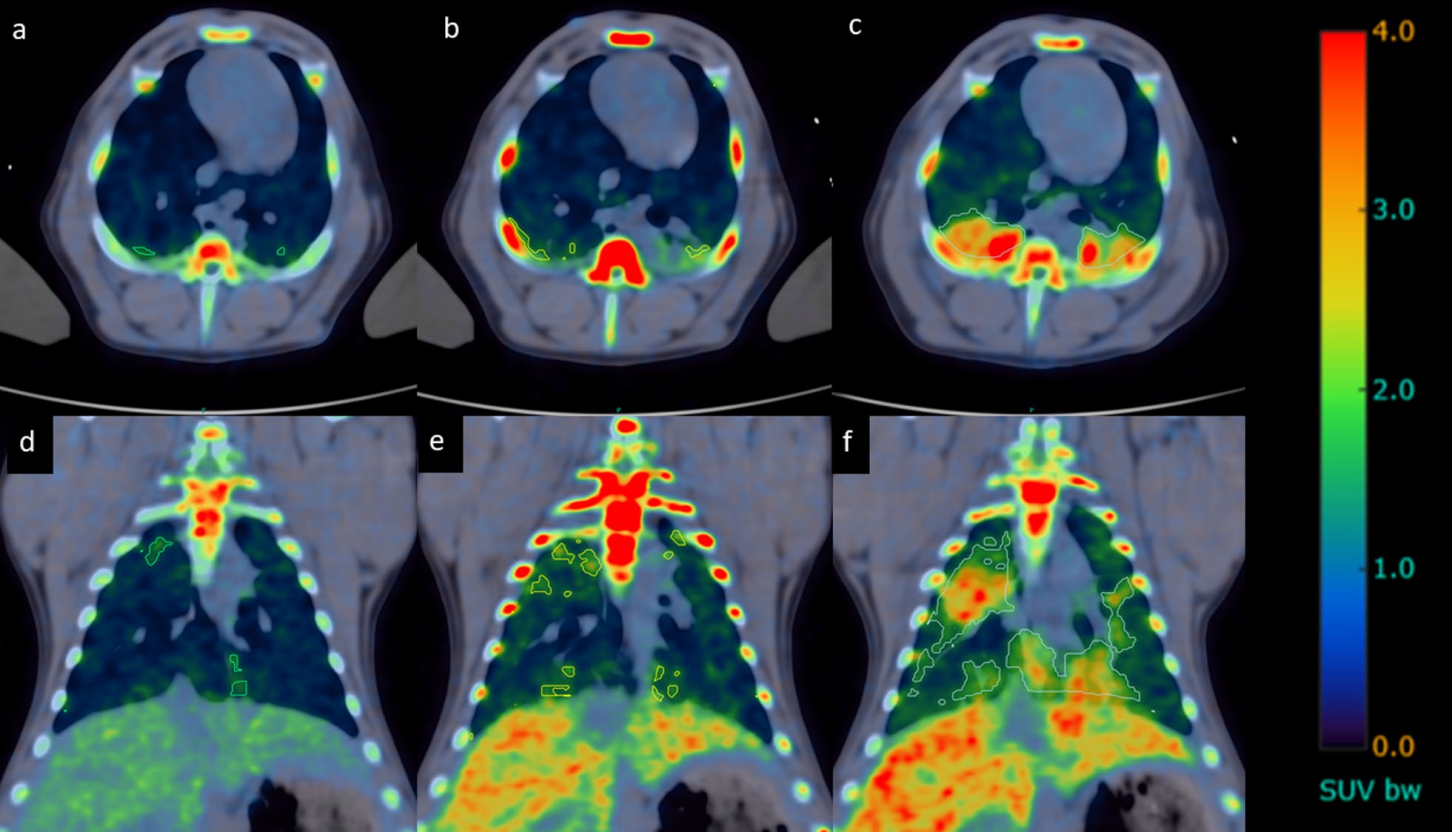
Upper row: trans-axial PET–CT images over the lungs: a − 1 h, b 2 h, c 4 h. Lower row: coronal PET–CT images. d − 1 h, e 2 h, f 4 h. Both the volume of affected lung and the mean SUV of the affected area increase over time, causing the total inflammatory volume of the lungs (TIL) to increase markedly
